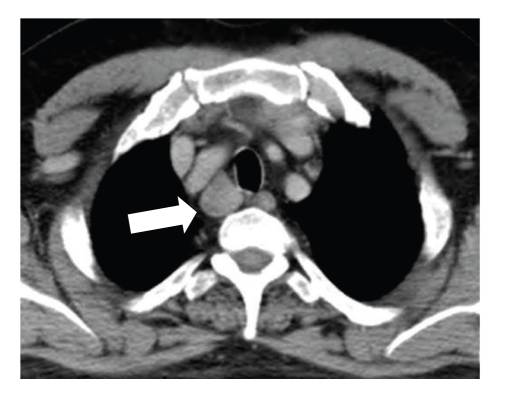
CT neck showing large right sided neck lesion.
